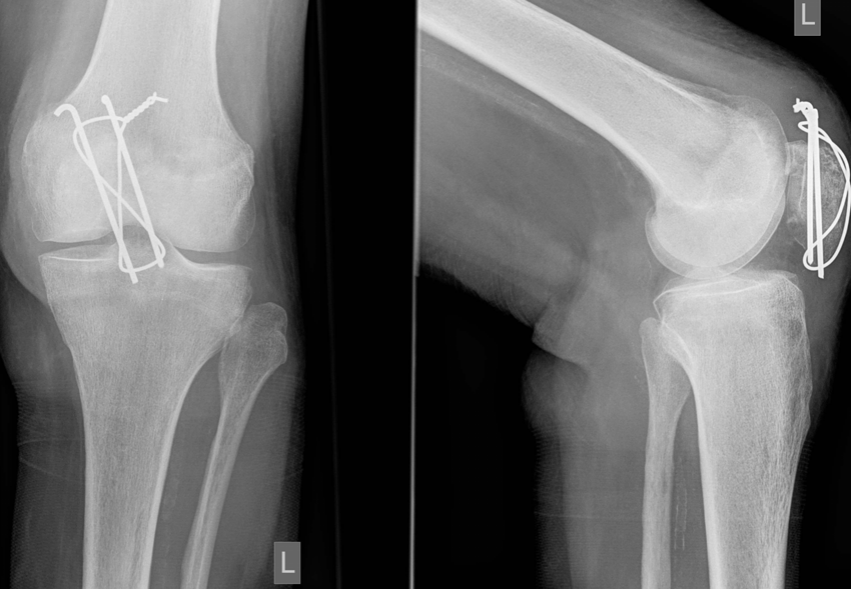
Anteroposterior and lateral radiographs of left knee 3 months after patellar fracture and cerclage wiring (K-wires and wiring perpendicular to fracture line).
